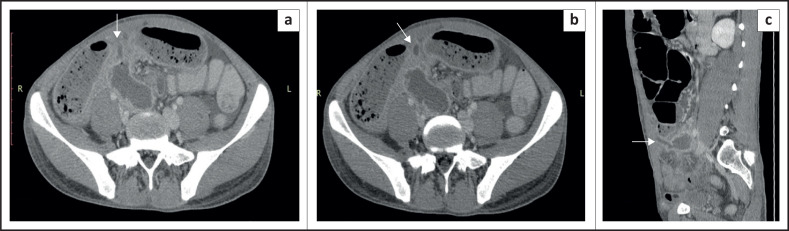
Contrast enhanced computed tomography in the axial (a, b) and sagittal (c) planes revealed a Meckel’s diverticulum (white arrows) with wall thickening and enhancement, surrounded by fat inflammation suggestive of Meckel’s diverticulitis.
